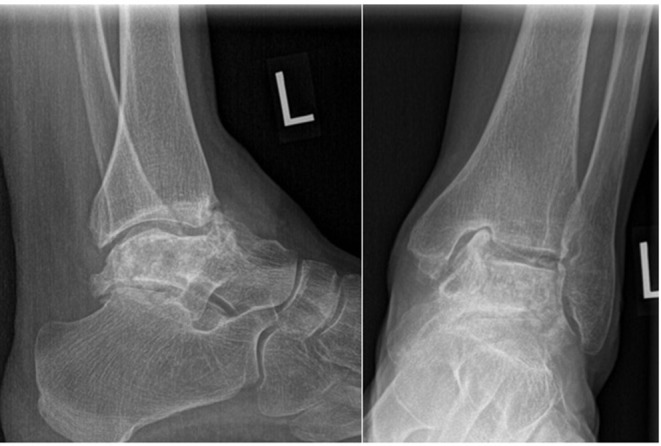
Pre-operative left ankle radiographs. Note the severe collapse since the previous radiograph and groove in the medial corner of the tibial plafond.
